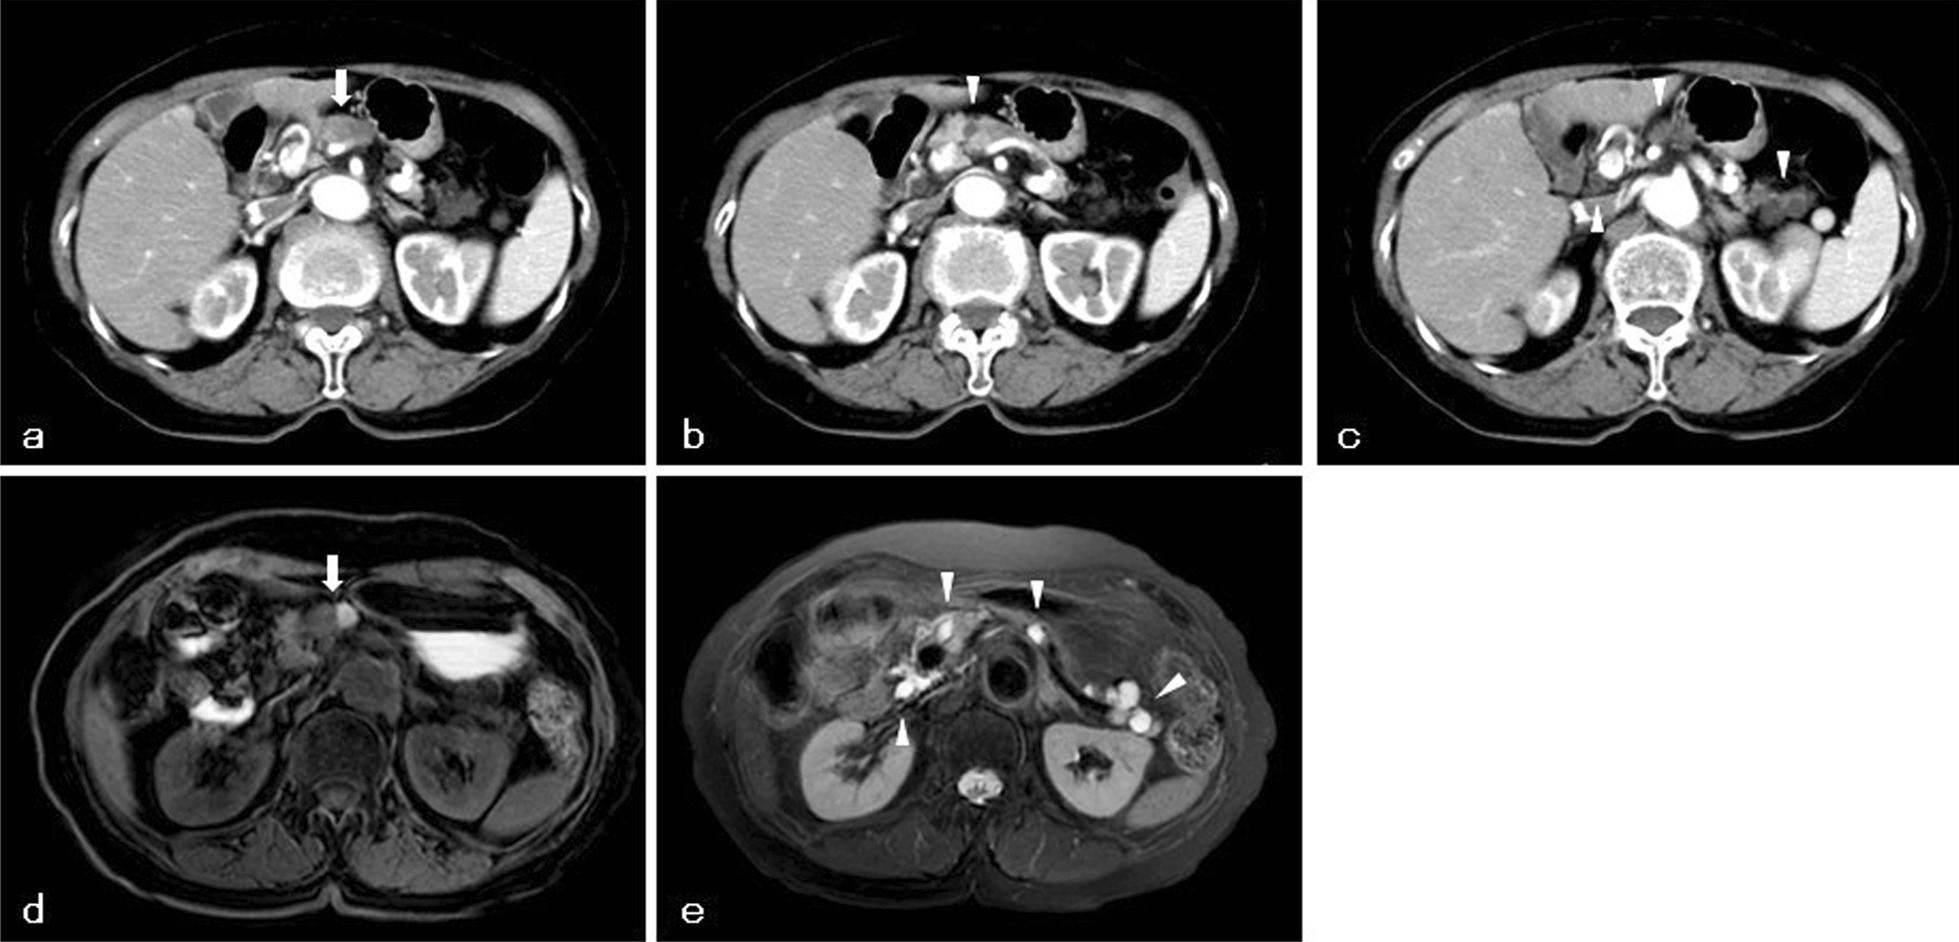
Enhanced computed tomography and magnetic resonance imaging images. Enhanced computed tomography revealed a poorly enhanced nodule (white arrow) on the pancreatic body (a) and a cystic lesion (white arrowhead) adjacent to the tumor (b). Additionally, several cystic lesions (white arrowheads) were detected throughout the pancreas (c). Magnetic resonance imaging revealed a tumor with a partially high-intensity area (white arrow) on the pancreatic body (d). Further, several cystic lesions (white arrowheads) were detected throughout the pancreas on T2-weighted magnetic resonance imaging (e)
